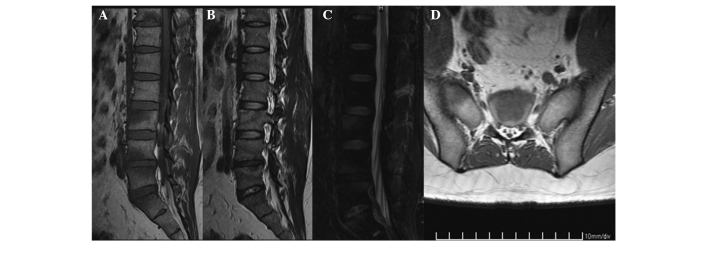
Sagittal magnetic resonance imaging (MRI) scans of the lumbar spine revealed diffused inflammatory bone changes in the T11, L3–L5 and S1 vertebral bodies that were (A) hypointense on the T1-weighted image, (B) hyperintense on the T2-weighted image and (C) hyperintense on the fat-saturated T2-fast spin-echo sequences. (D) Axial MRI of the sacroiliac joints demonstrated edema around the bilateral ala of the sacrum on the T1-weighted image.
